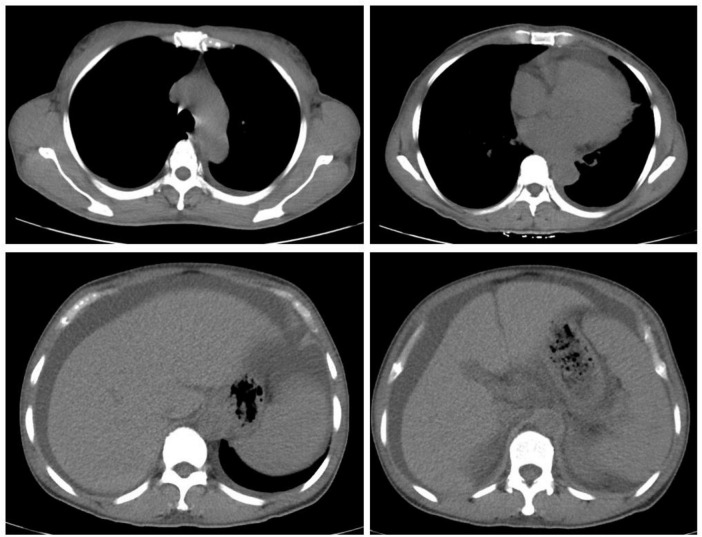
CT revealed hepatosplenomegaly and multiple serous effusion. CT, computed tomography.
